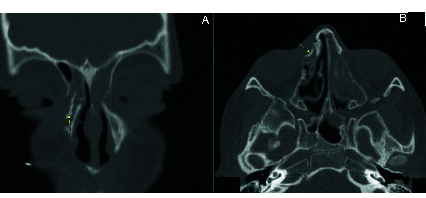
Preoperative orbital and paranasal sinuses on CT. Non-contrast-enhanced bone window CT scan, coronal (A) and axial (B) views, showing a hyperdense lesion in the lacrimal sac, with a Hounsfield scale
